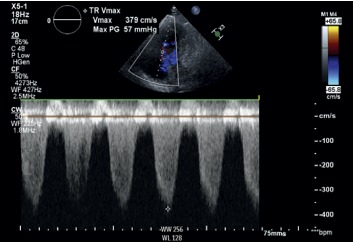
Continuous wave-Doppler study showing tricuspid insufficiency with an estimate peak pressure gradient of 57 mm Hg.
